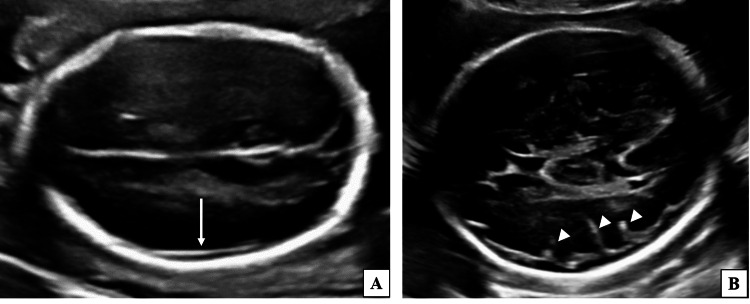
Two-dimensional ultrasound grey-scale images (axial sections) of the fetal head demonstrating the appearance of convexity sulci. (A) Fetus of 21 weeks 3 days gestational age showing a smooth lateral brain surface without any sulcal indentations (long white arrow). (B) Fetus of 28 weeks 5 days gestational age demonstrating multiple indentations on the lateral brain surface (white arrowheads) suggestive of convexity sulci.
